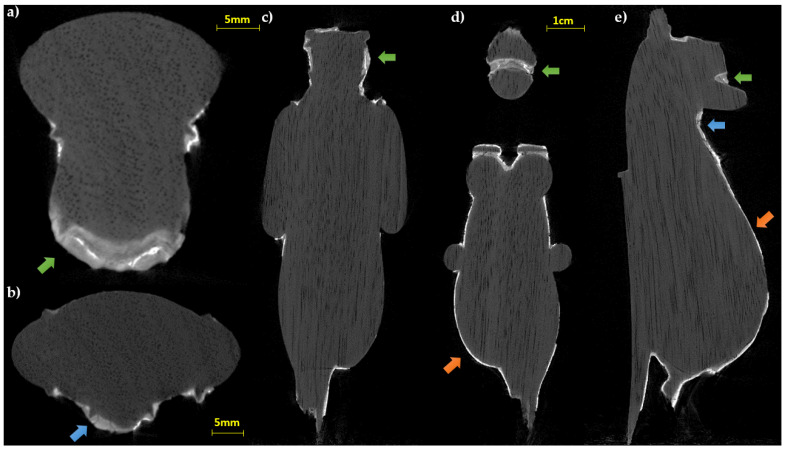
CT slices of the statuette: (a,b) horizontal sections; (c–e) longitudinal slices. In all the sections the wood structure (vessels and growing rings) is visible (green arrows: preparation layer in the face area; blue arrows: fractures inside the preparation layer; orange arrows: thin preparation material for the gold leaf adhesion).
